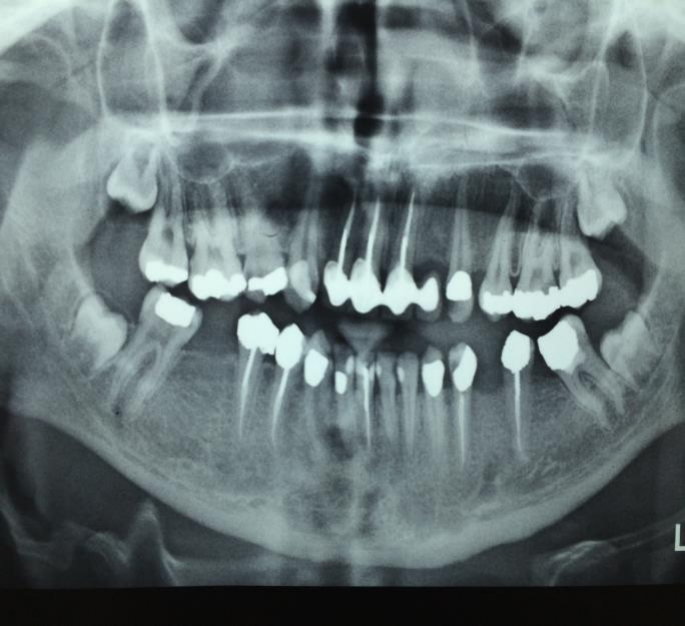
In panoramic radiography, because of hypoplastic enamel and poor oral hygine, all of teeth had feeling or need to endodontic treatments
